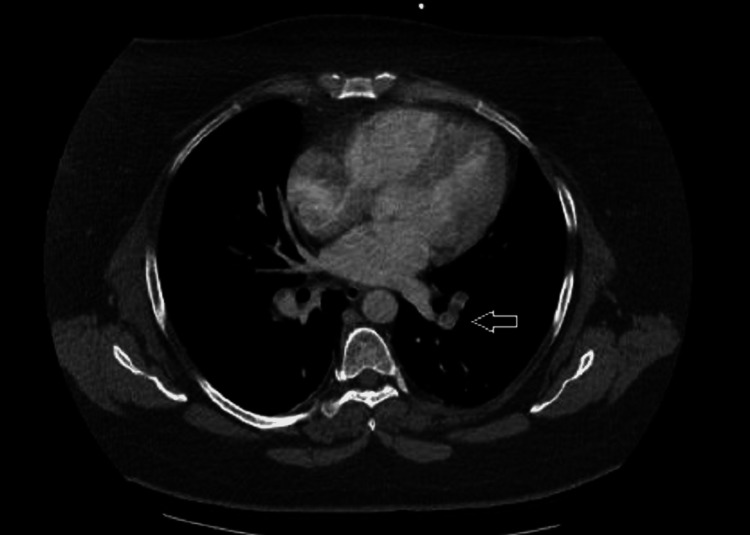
CT chest angiogram demonstrating bilateral pulmonary embolism (white arrow) CT: computed tomography
